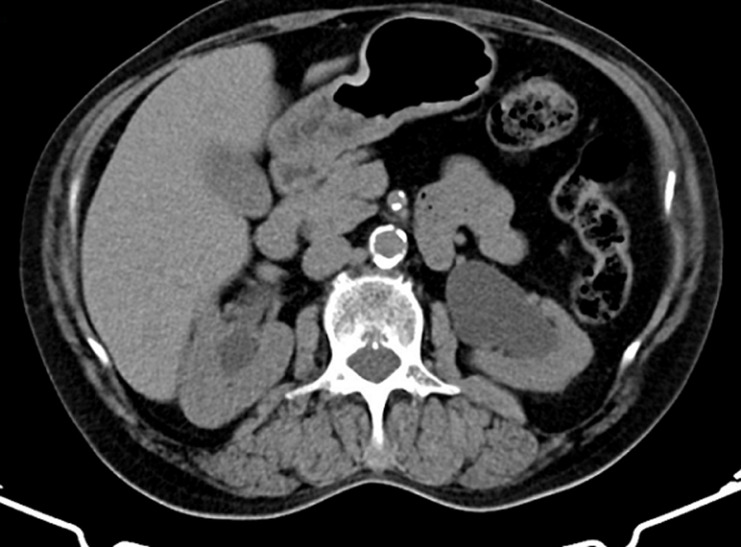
preoperative CT imaging of the abdomen
